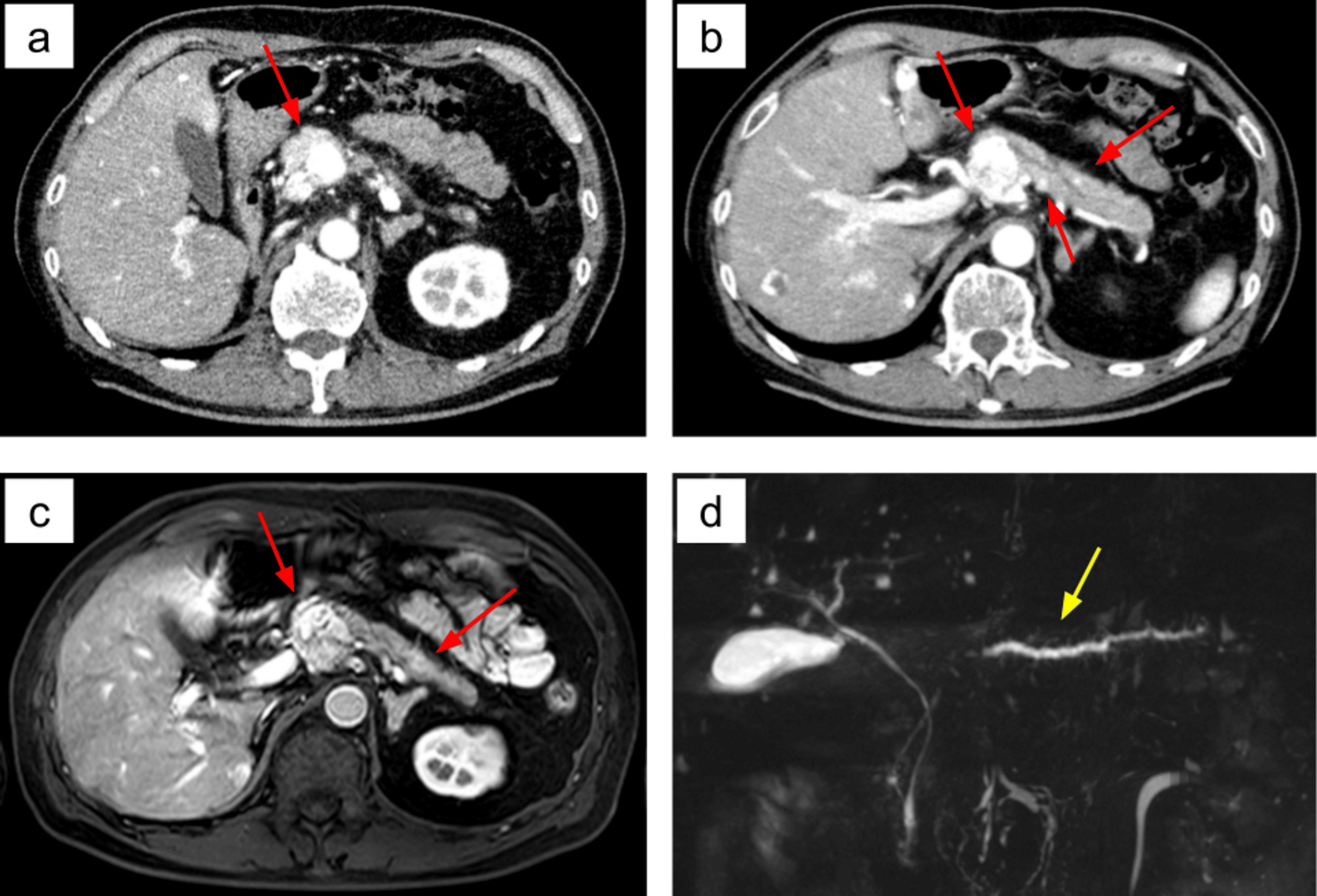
Preoperative images of the pancreatic tumors CT (a, b) and MRI (c, d) showed multiple hypervascular, early-enhancing tumors in the pancreas (red arrow). The largest of these, measuring 37 mm, was located in the pancreatic head (a, b, c). Smaller, intensely enhancing lesions were also present in the body and tail (b, c). MRCP demonstrated mild dilation of the main pancreatic duct in the body and tail (yellow arrow).
